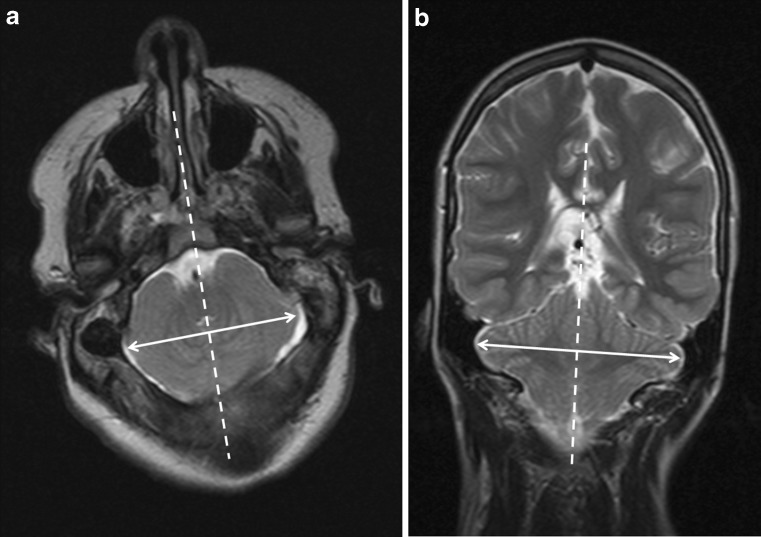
a Axial T2-weighted brain MR image of a 16-year-old child with open spinal dysraphism and Chiari II malformation. The *arrow* indicates axial cerebellar width; b coronal T2-weighted brain MR image of a 13-year-old child with open spinal dysraphism and Chiari II malformation. The *arrow* indicates coronal cerebellar width
